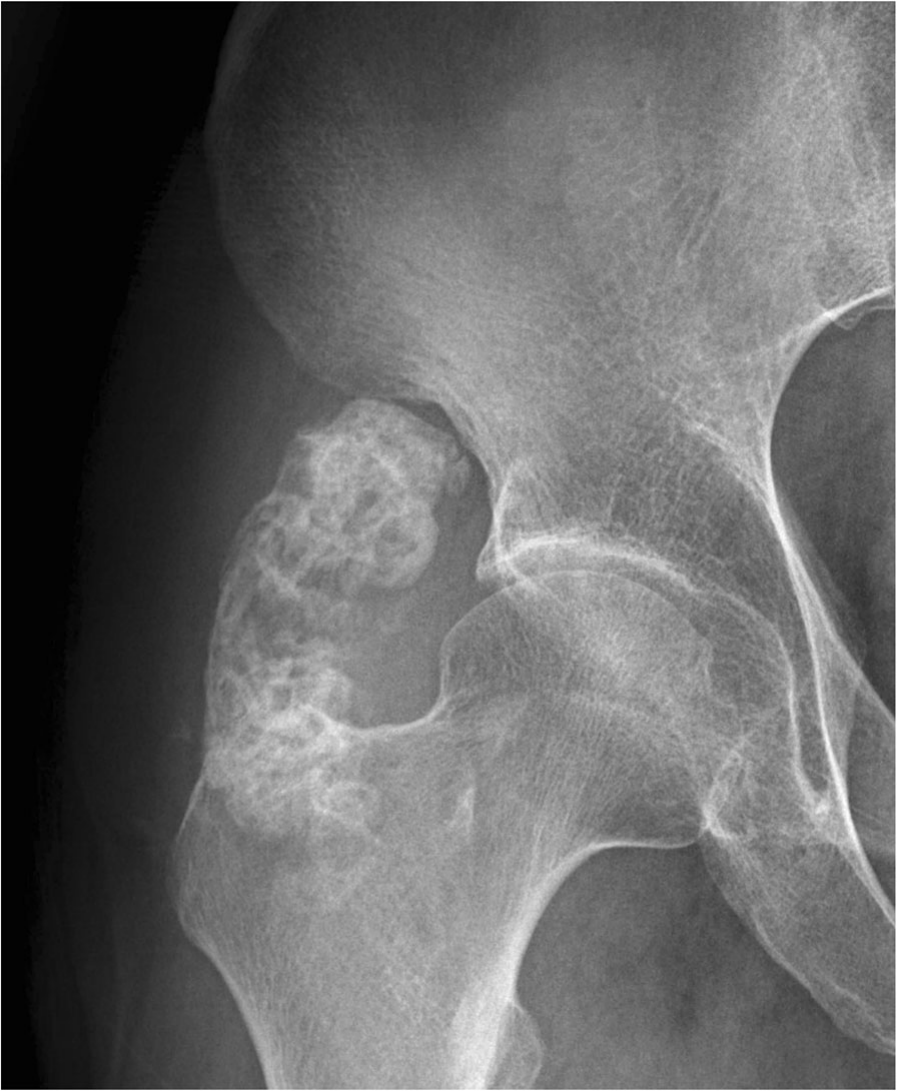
X-ray revealed extensive damage and cortex destruction of the right remi inferior ossis pubis
